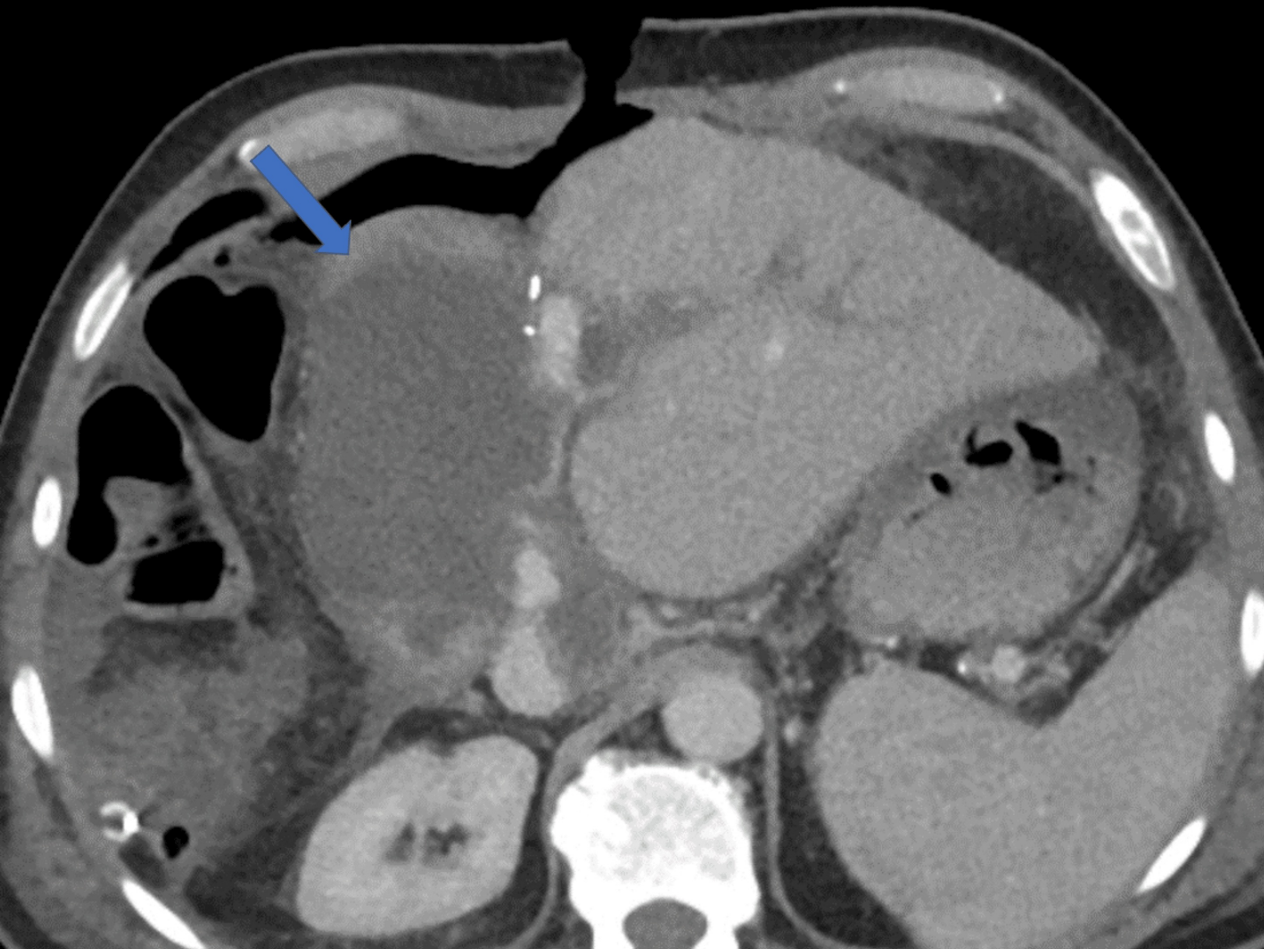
Postoperative CT imaging A CT scan demonstrates a collection (arrow) compressing the hepatico-jejunostomy, resulting in a bilio-cutaneous fistula. The collection appears not amenable for drainage, as it is lying under the small bowel loop carrying the hepatico-jejunostomy. CT: computed tomography
